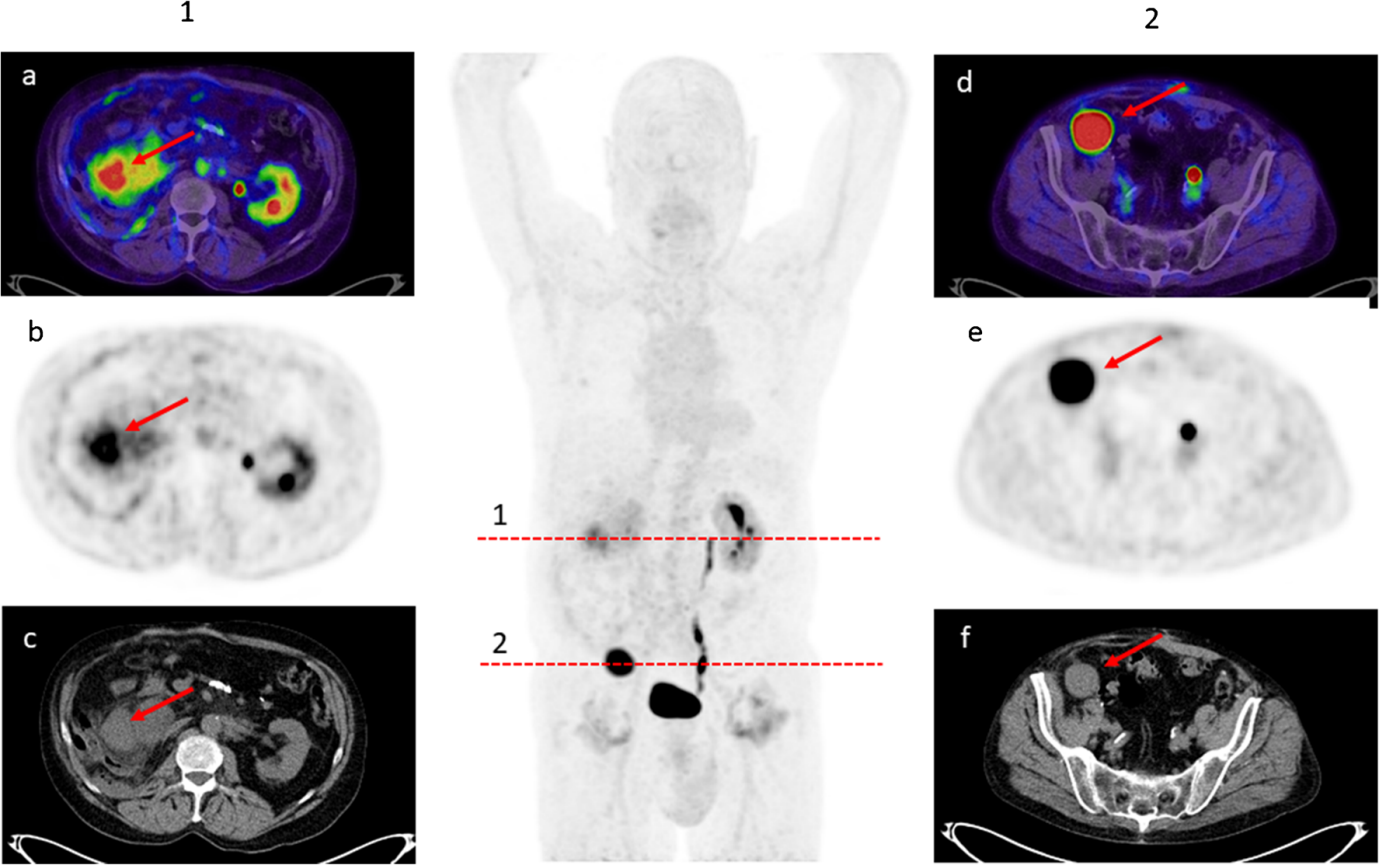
69-year-old patient with a bi-focal relapse of a liposarcoma detected by ^68^Ga-FAPI PET/CT (a, d FAPI PET/CT Dx; b, e FAPI-PET Dx; c, f CT Dx). (1) SUV_max_ 8.98 SUV_mean_ 4.60 (2) SUV_max_ 19.39 SUV_mean_ 13.18
